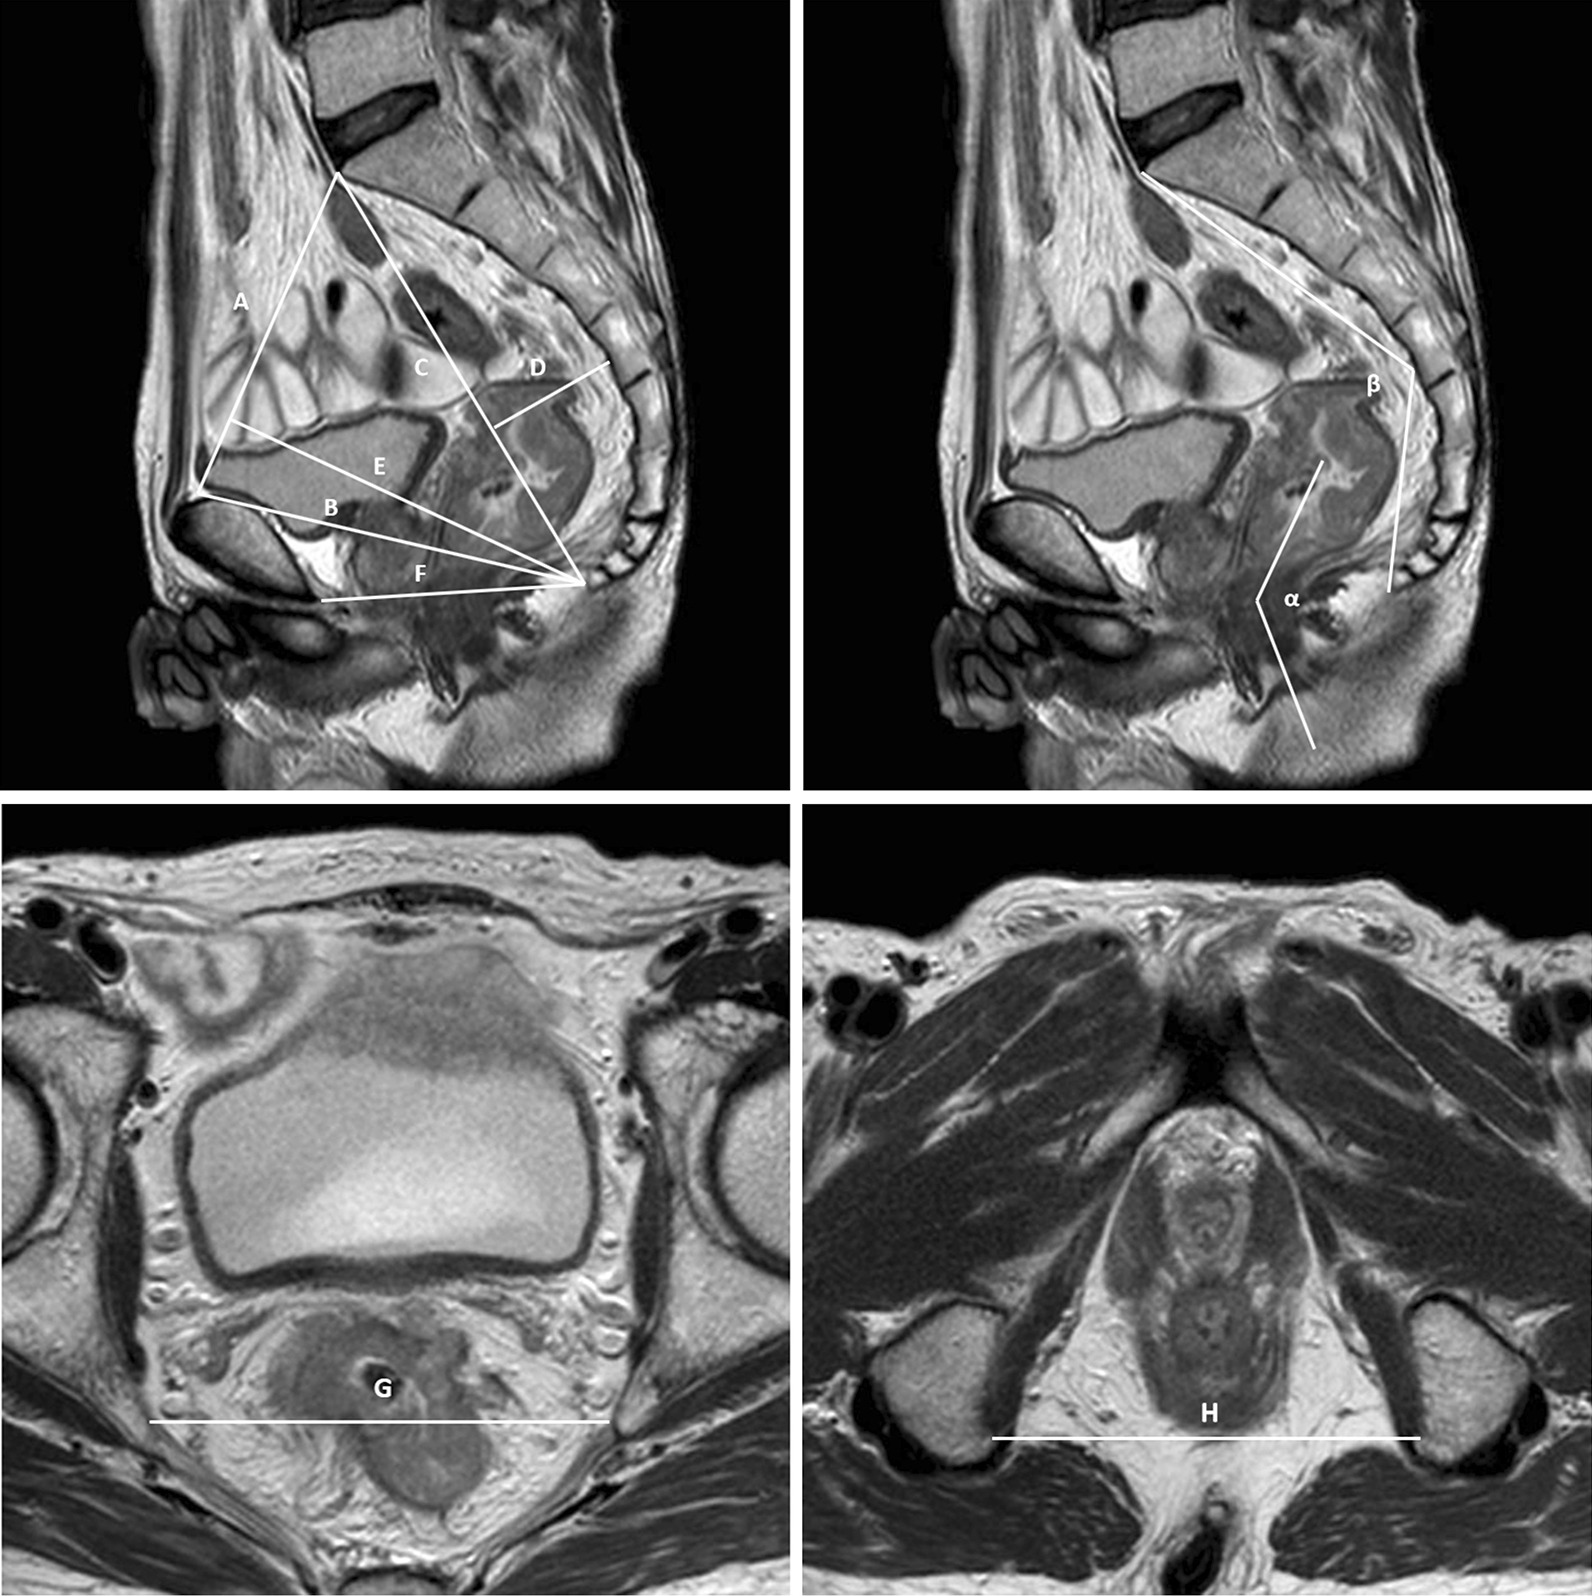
MRI-based pelvimetry. A: pelvic inlet; B: pubococcygeal distance; C: pelvic length; D: sacral depth; E: pelvic depth; F: pelvic outlet; G: interspinous distance; H: intertuberous distance; α: anorectal angle; β: sacrococcyx angle
